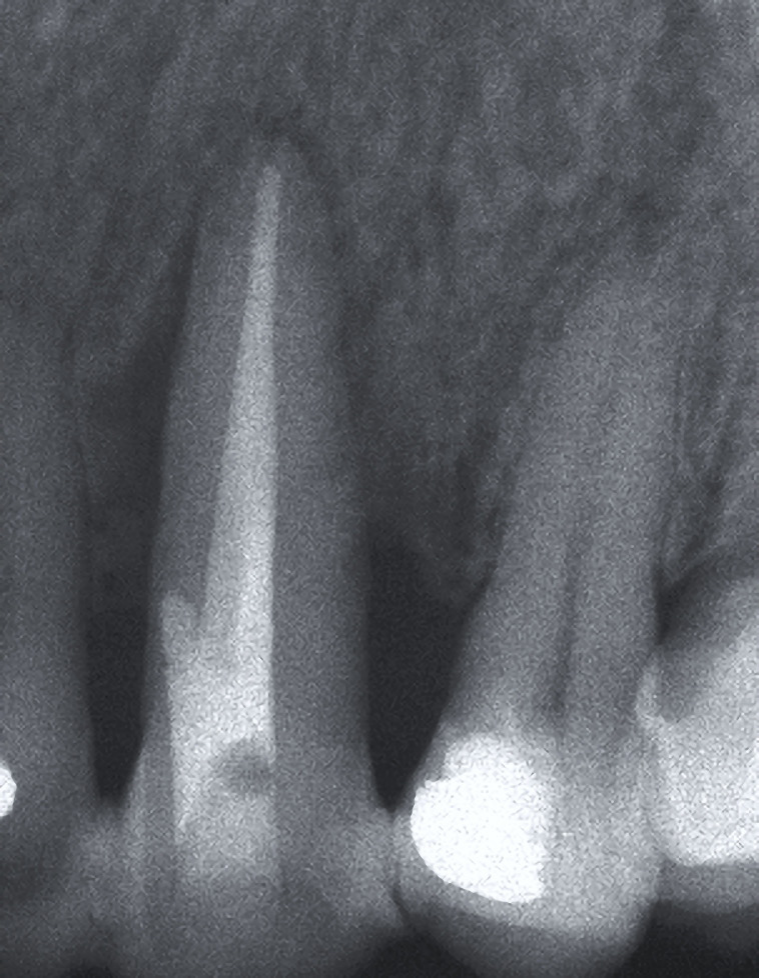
Radiograph showing a moderate decrease in periapical and lateral radiolucency after 6 months.
